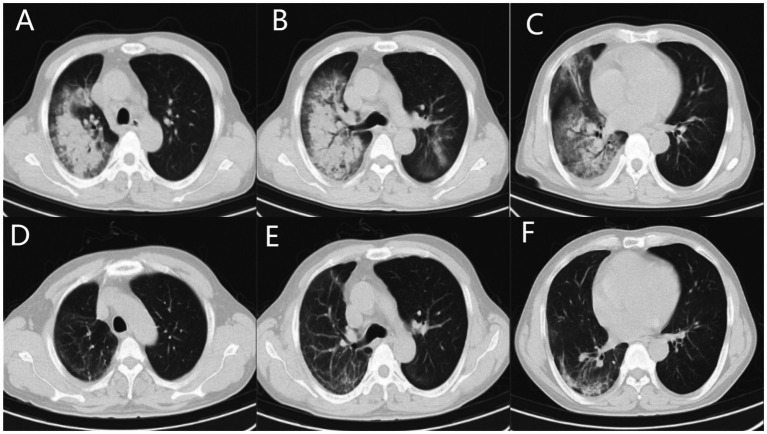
Chest computed tomography (CT) of the patient: The initial chest CT on admission showed dual pneumoniaous lesions with minimal right pleural effusion (A–C). After 15 days of omadacycline treatment, the chest CT showed a reduction in pulmonary consolidation and pleural effusion (D–F).
